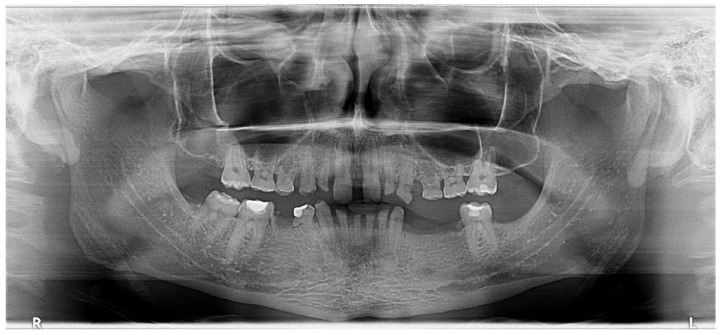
OPG. Radiographic initial situation of the patient. The radiograph reveals the presence of 7 permanent teeth on the dental arches.
